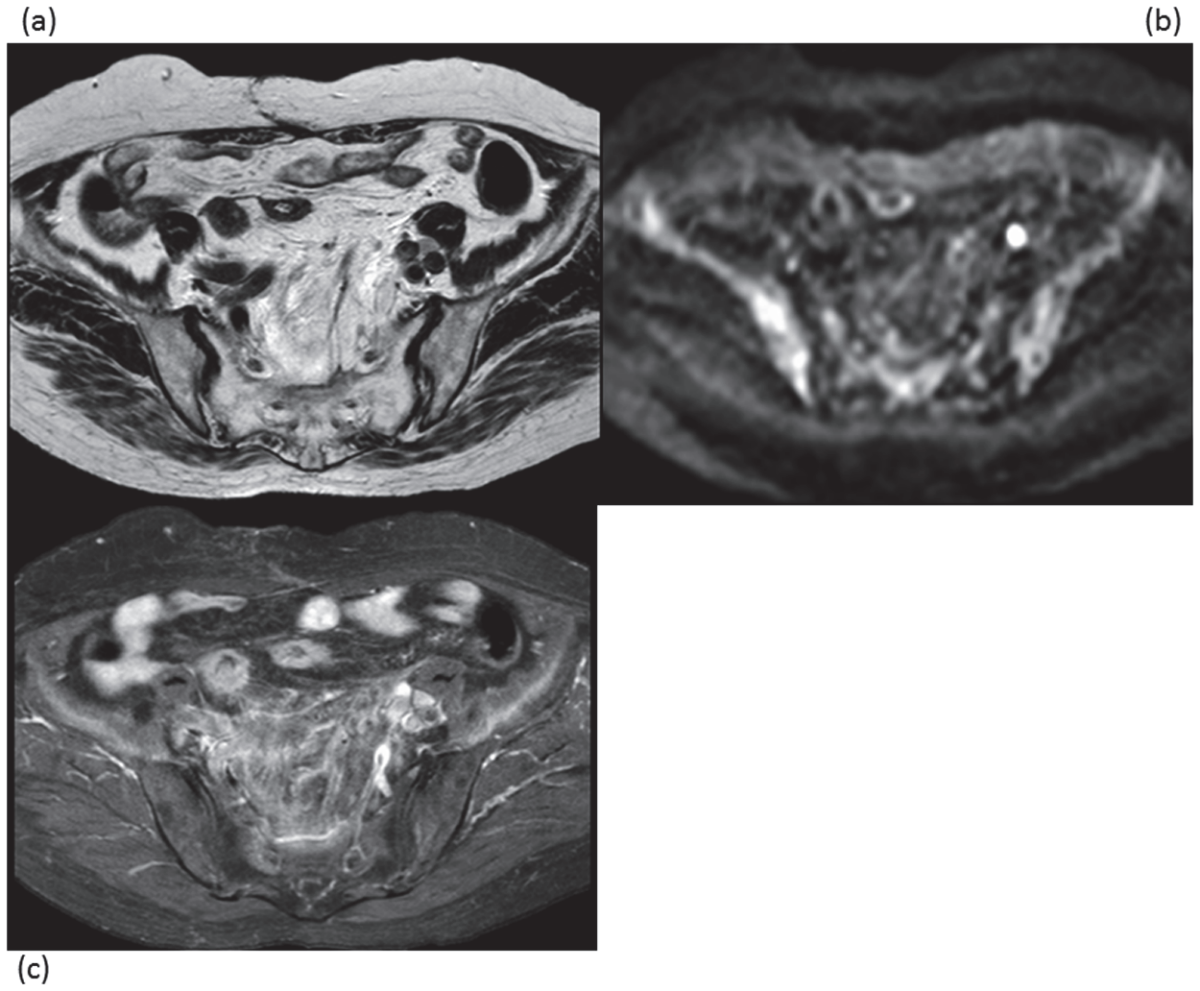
A 65-year-old woman with intrapelvic lymph node recurrence 12 months after surgery for endometrial cancer. (a) Axial T2-weighted MRI shows a 9-mm left external iliac LN (arrow). This equivocal finding for lymph node recurrence was assigned a score of 3 for protocol A. (b) Axial DWI shows a focal hyperintense spot in the left external iliac area (arrow), corresponding to the node seen in (a), being suspicious for lymph node recurrence. The score assigned for protocol B was 4. (c) Axial contrast-enhanced MRI shows slight ring-like enhancement of the same node (arrow), suggesting lymph node recurrence. The score assigned for protocol C was 4. Follow-up MRI showed an increase in the size of this node, confirming lymph node recurrence.
